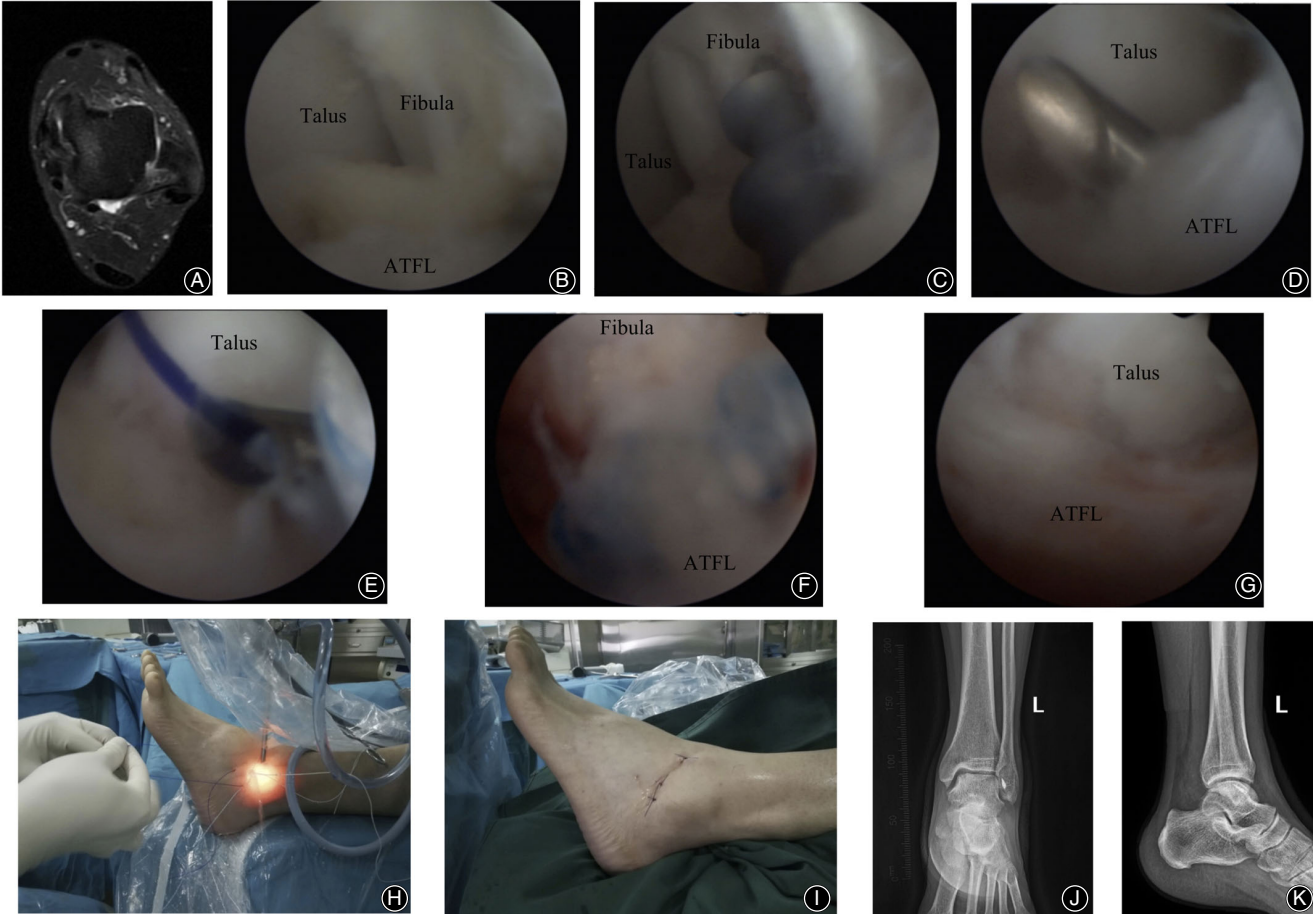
A 32‐year‐old male with recurrent sprain of the left ankle with unstable walking for 18 months. The symptoms were not relieved after 12 months of regular conservative treatment. Arthroscopic anatomical repair of ATFL was performed. (A) Preoperative MRI plain scan of the left ankle suggested that continuity of ATFL was interrupted. (B) Arthroscopic exploration showed horizontal avulsion of ATFL from the fibular stop point and relaxation of ATFL without tension. (C) The footprint region of the fibula was observed under arthroscopy. After freshening, a double wire anchor with a diameter of 3.5 mm was inserted. (D) The ATFL was sutured with a suture hook under arthroscopy. (E) Under arthroscopy, the anchor sutural line was guided through the ATFL after the PDS sutural line was used to pass through the suture hook. (F) Fibular side of the ATFL was sutured with a knot pusher. (G) The ATFL returned to normal tension and strength after suture under arthroscopy. (H) During the operation, the PDS sutural line was used to guide the anchor wire through the external phase. (I) The surgical approach after suture. (J, K) Anterior–posterior and lateral X‐ray films of the ankle after surgery.
